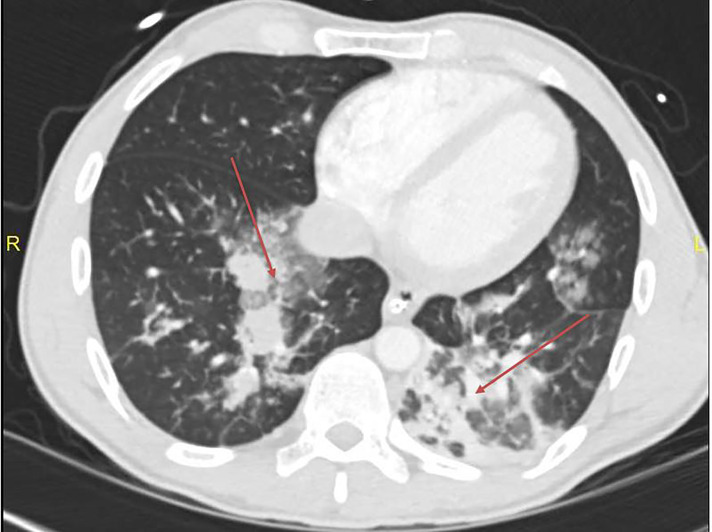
Computed tomography showing extensive ground-glass opacities (arrows) in multiple lung fields concerning for inflammatory or infectious etiology.
